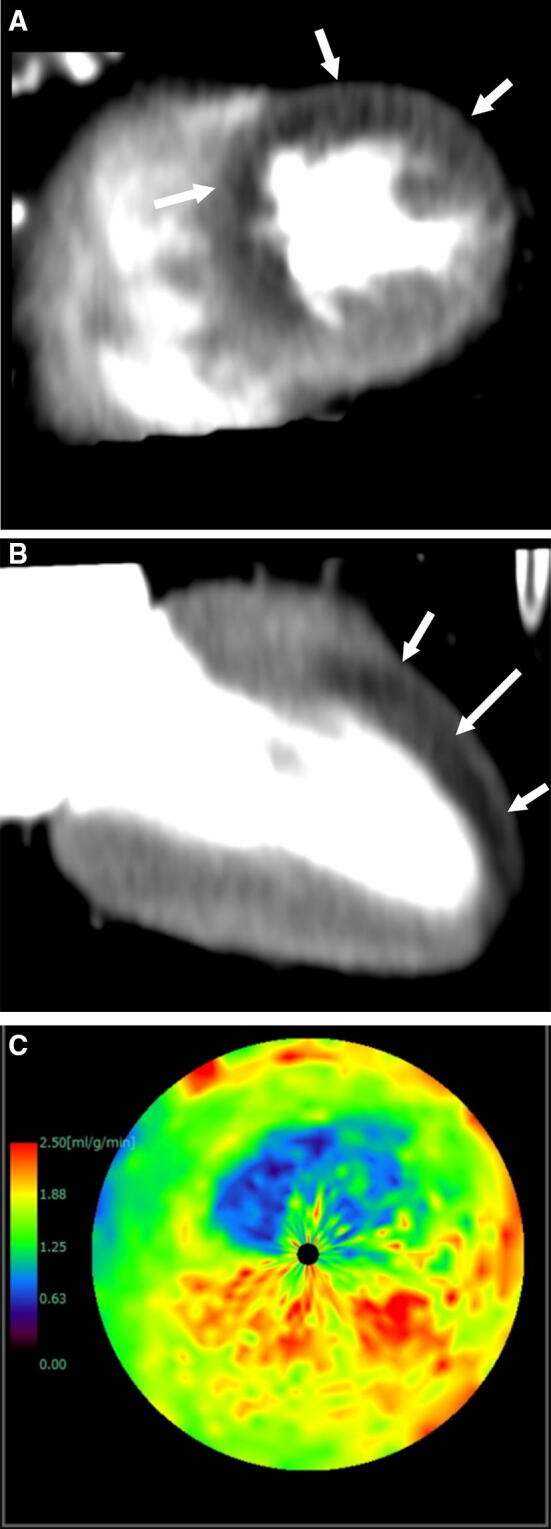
Dynamic CTP scans for single-vessel disease in an 87-year-old man with chest pain. He had hypertension and a history of smoking. Short-axis (a) and two-chamber (b) grayscale stress dynamic CTP images showing a subendocardial perfusion defect in the anterolateral, anterior, and anteroseptal segments (arrows). c A CT-MBF color-coded image shows that the MBF in the ischemic myocardium is lower than in the remote myocardium. *CTP* computed tomography perfusion, *CT-MBF* computed tomography derived-myocardial blood flow
